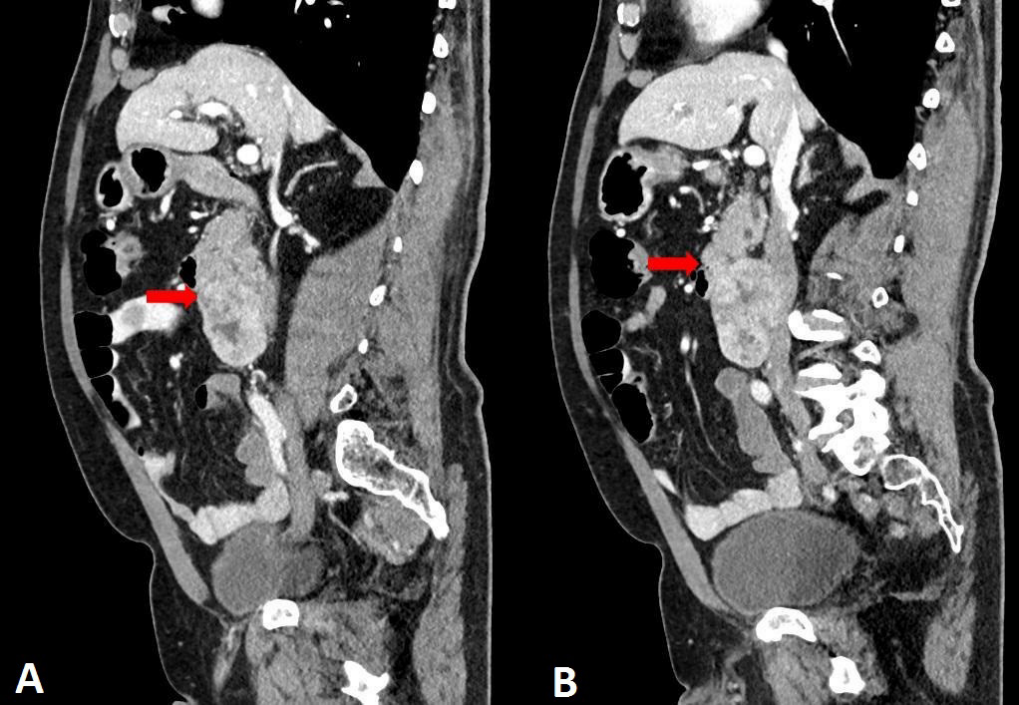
Abdominal computed tomography scan, sagittal views. Red arrows demonstrate a large retroperitoneal mass (images A and B) located inferior to the uncinate process of the pancreas, adjacent to the posterior wall of the third portion of the duodenum (causing anterior deviation), and in close proximity to the right lateral wall of the aorta, with no clear cleavage plane from the inferior vena cava.
